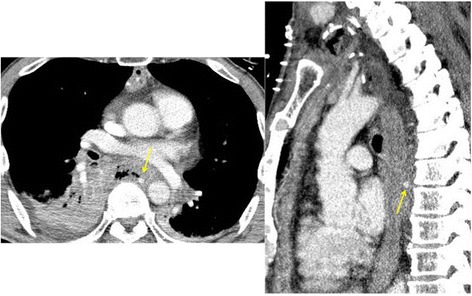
Enhanced computed tomography on hemoptysis revealed contrast medium extravasation, indicating a pseudoaneurysm (*arrow*) at the mediastinum
